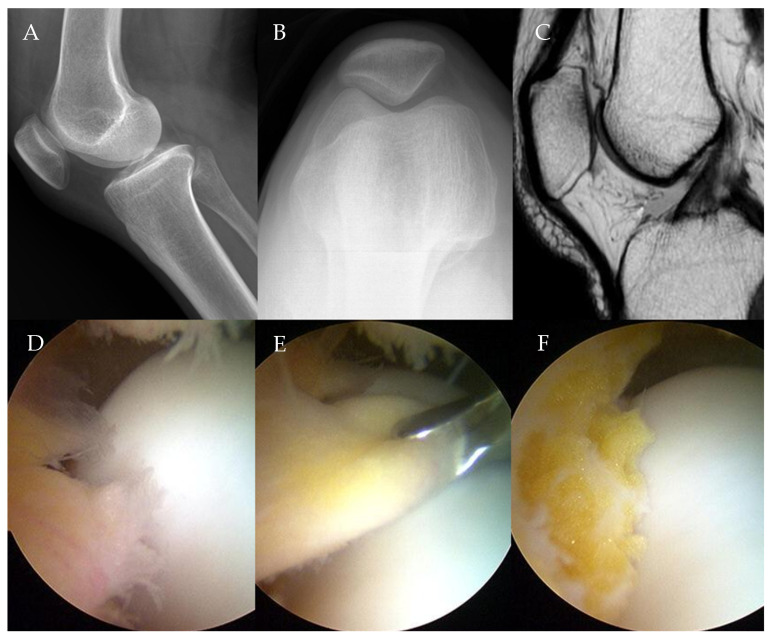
A 46-year-old female patient complaining of anterior knee pain of VAS 6 points that did not improve despite conservative treatment for more than 6 months. (A) Lateral knee radiograph showing an Insall–Salvati ratio of 0.98. (B) Merchant view showing K-L grade 0. (C) MRI showing infrapatellar fat pad volume of 656.24 mm^2^ and inflammatory and edematous changes. (D) Arthroscopic finding showing infrapatellar fat pad impingement. (E) Resection of the infrapatellar fat pad with a basket. (F) Infrapatellar fat pad after arthroscopic subtotal resection.
